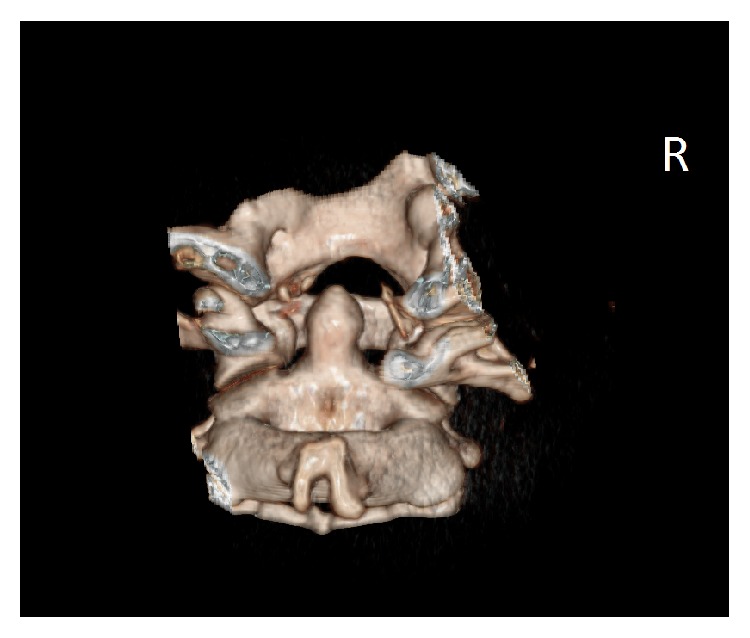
Three-dimensional CT reconstruction image shows avulsion fracture of the right occipital condyle (back view).
